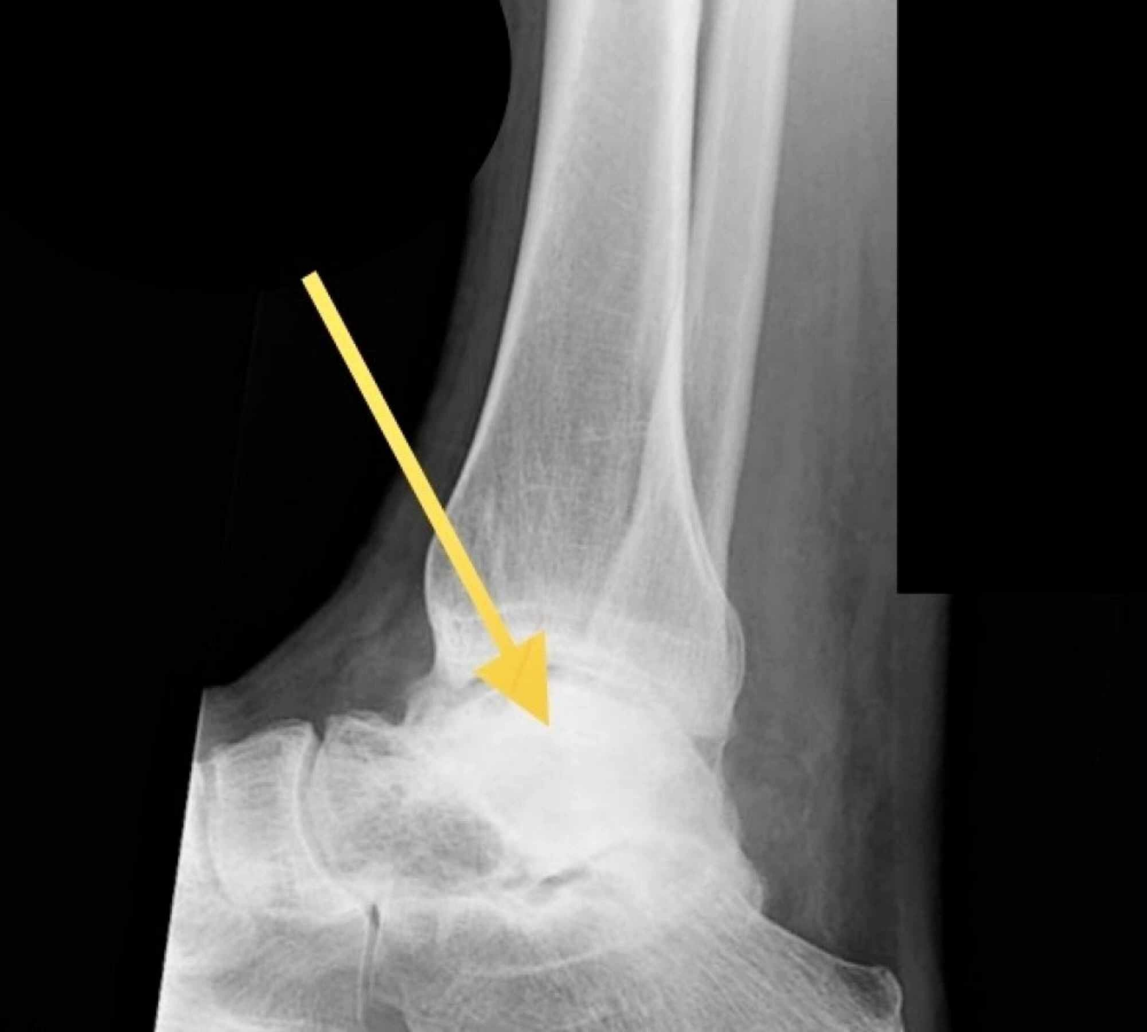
A lateral radiograph of the right ankle demonstrating signs of avascular necrosis of the talus (arrow)
